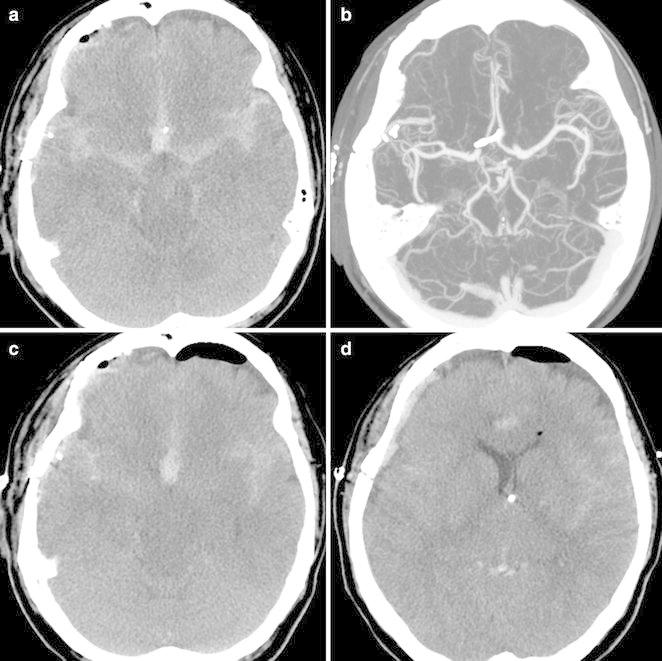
On post-operative Day 3, (a, b) head CT scan and CT angiography revealed no evidence of re-bleeding, hydrocephalus or vasospasm but showed an elongated midbrain. On post-operative Day 5 (c, d), head CT scan showed small lateral ventricles and effacement of the cisterns
